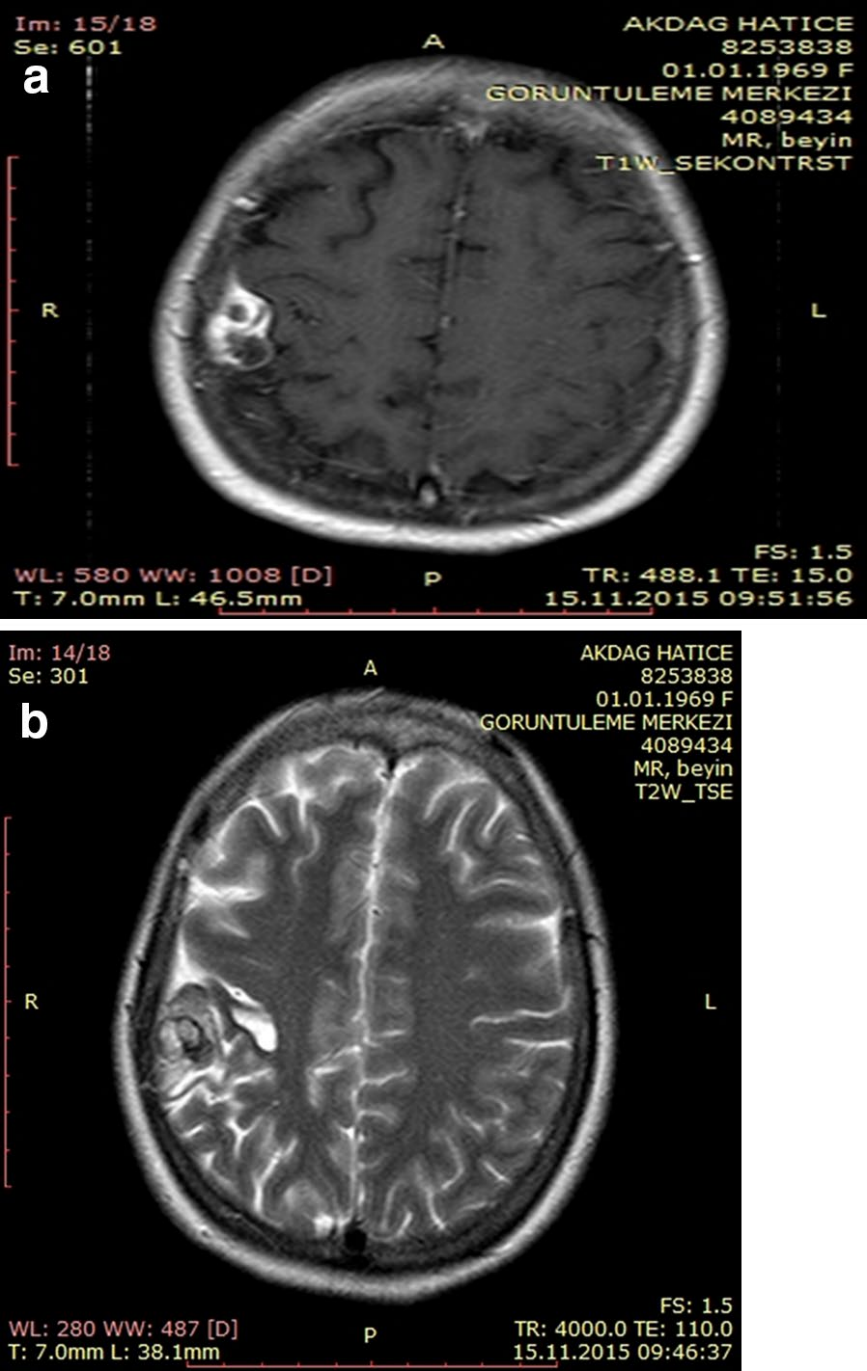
a, b Preoperative cranial MRI of the patient. T1A and T2A sections show a clearly contrast-enhanced hypo- and hyper-intense lesion at the right posterior parietal vertex
